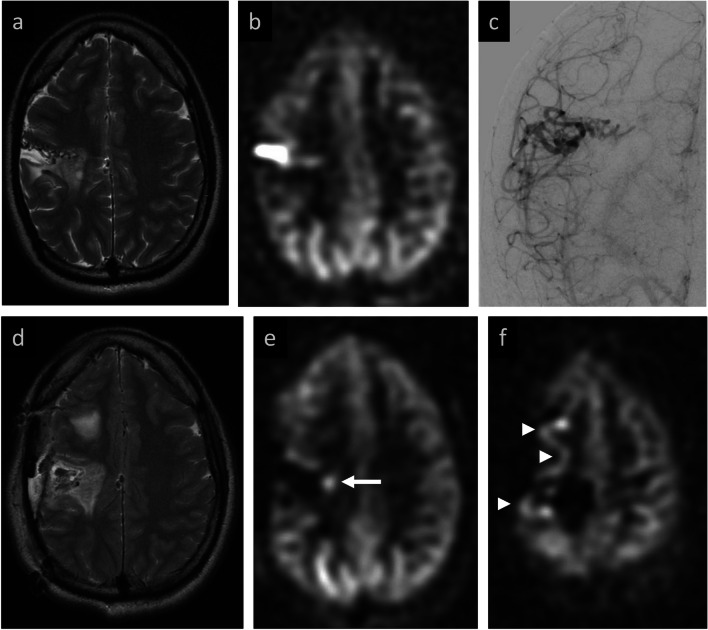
Example of inter-rater disagreement in evaluation of recurrent AVM. Baseline MRI: tangle of vessels in right lateral frontal lobe adjacent to an old resection cavity (a) with corresponding marked ASL signal suggestive of ongoing AV shunting (b). Baseline DSA: frontal projection confirms AVM nidus with early venous drainage (c). Post-resection MRI: immediate post-operative changes without visible nidus (d). Mild marginal ASL signal (white arrow, e) was attributed to post-surgical change by both readers, but curvilinear ASL signal superiorly (white arrowheads, f) was thought to reflect residual AV shunting by one reader. On consensus review, signal was felt to be gyriform and without co-localizing flow voids, ultimately attributed to clinically-confirmed seizure, rather than residual shunting. Post-resection DSA: confirmed AVM obliteration (not shown)
